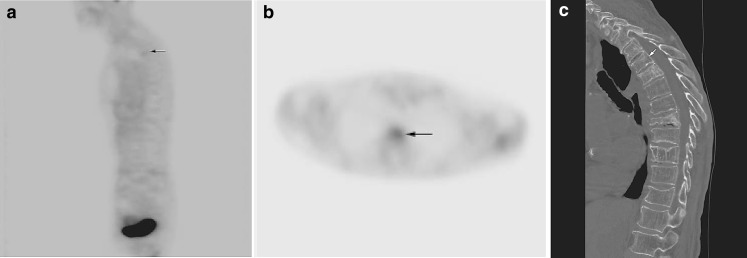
a Benign subacute compression fractures in a patient without history of malignancy. Sagittal FDG-PET image demonstrates mildly increased radiotracer uptake in the upper thoracic spine (SUV = 1.8; *arrow*). b Axial FDG-PET image at the level of T4 demonstrates mildly increased radiotracer uptake (*arrow*), consistent with subacute compression fracture. c Sagittal CT image demonstrates compression fracture of T4 (*arrow*) and multiple chronic osteoporotic compression fractures throughout the thoracic spine
